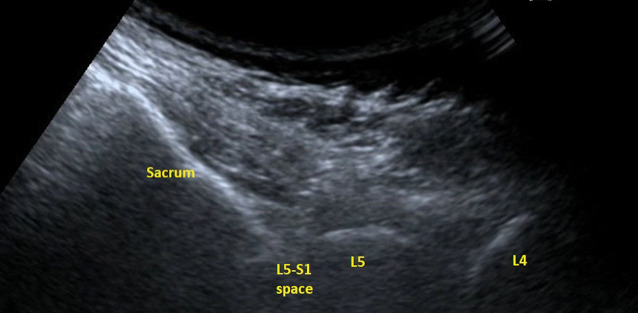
Ultrasound images of the L5/S1.
